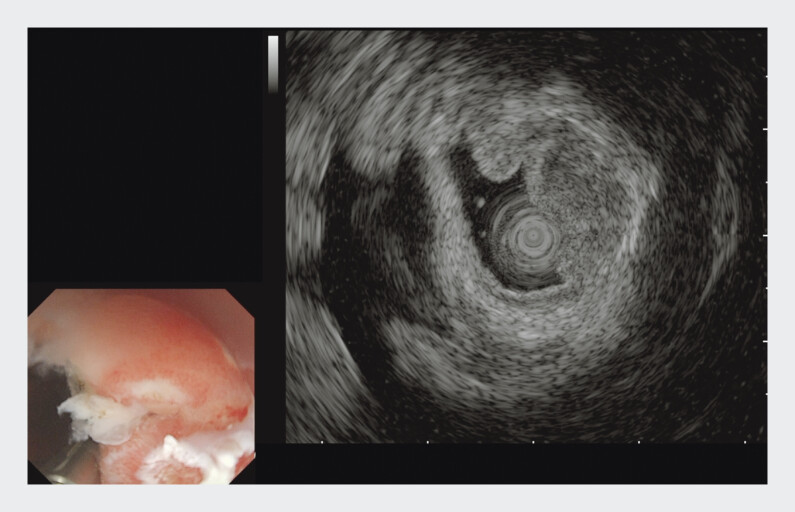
Endoscopic ultrasound revealed a suspected abscess at the esophagogastric junction.
